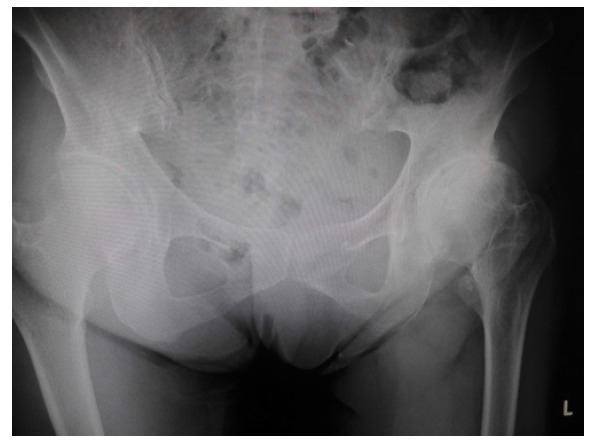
Roentgenogram of the hip joint shows a narrowing of the left joint space, which was diagnosed as left hip osteoarthritis.
